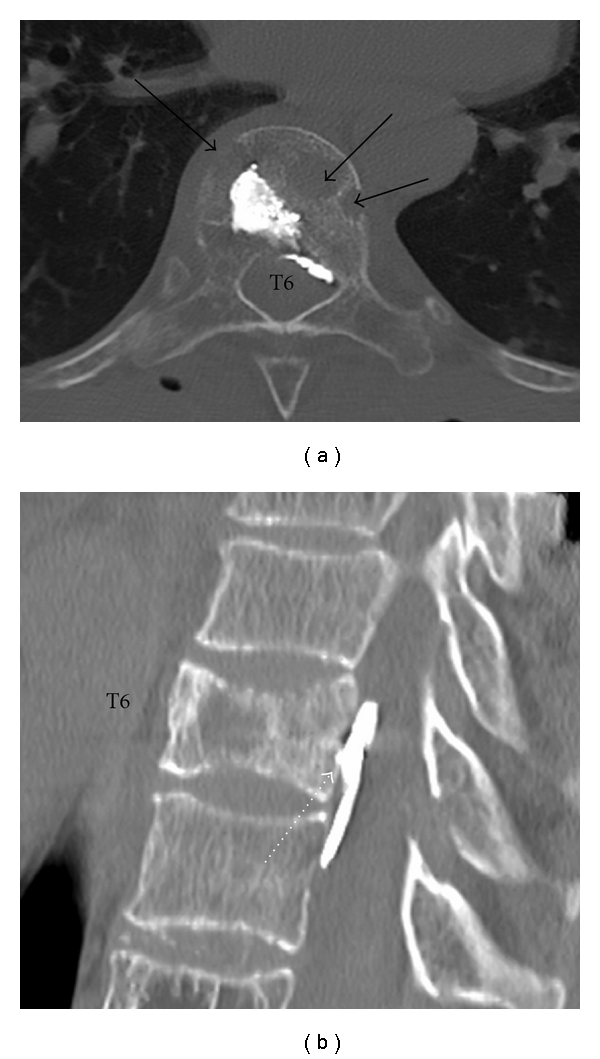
86-year-old woman with known multiple myeloma and pathological fracture of T6. During PV, posterior cement leak was noted soon after injection commenced; the procedure was abandoned. (a): Axial CT demonstrates permeative bone destruction of T6 (black arrows). Cement has extravasated posteriorly into the epidural space. (b) Sagittal CT demonstrates a thin collection of epidural cement anterior to the thecal sac (dashed white arrow). The patient remained asymptomatic initially and at followup and decompression was not required.
